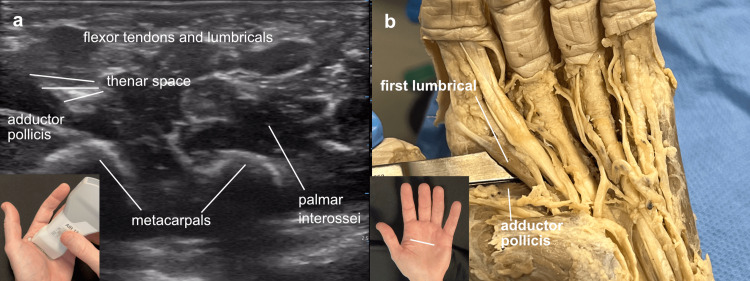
Thenar space Thenar space: (a) Ultrasound view of a left hand with the probe marker oriented to the left for easier correlation with the cadaver view, demonstrates the thenar space superficial to the adductor pollicis and deep to the first lumbrical, (b) the space is entered in the cadaver with blunt dissection between the adductor pollicis and the first lumbrical.
